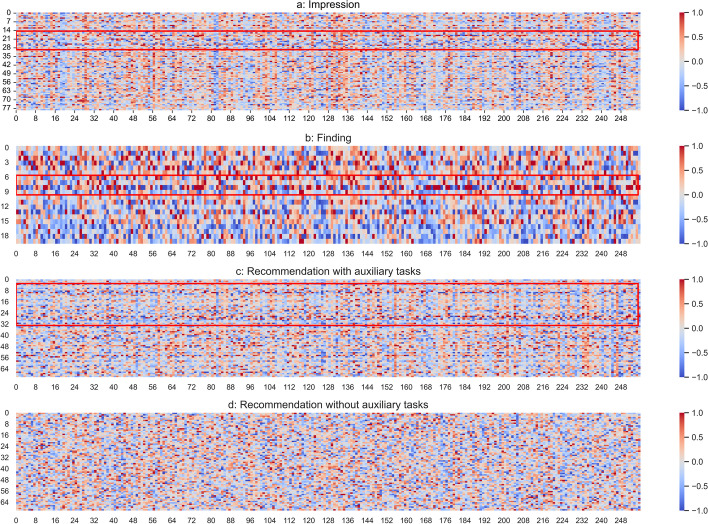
The visualization of an ultrasound report
